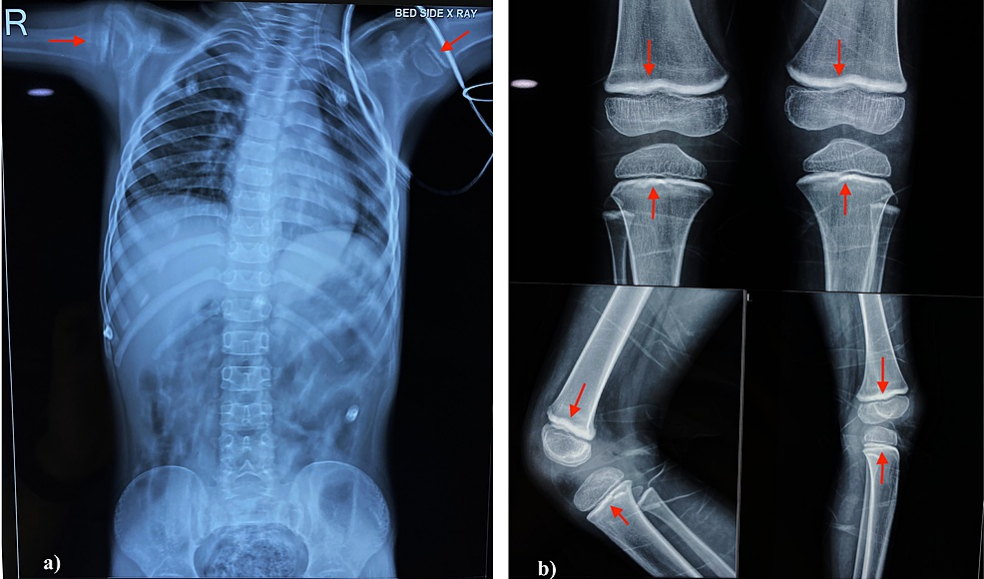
X-ray abdomen and long bones. (a) X-ray abdomen did not reveal any radio-opaque lead flecks. (b) X-ray of long bones (femur, tibia, humerus) showed lead lines at the metaphyseal ends.
